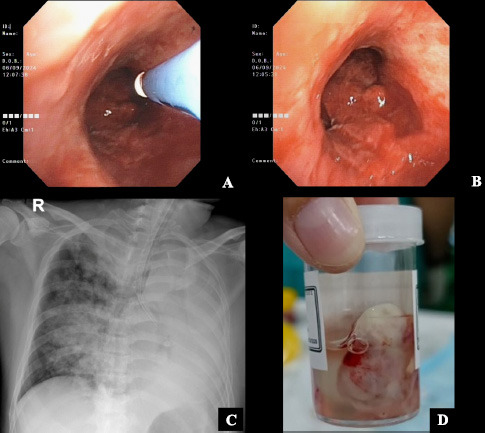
Intraoperative and postoperative findings following bronchoscopic intervention: (A) Argon plasma coagulation (APC) applied to control tumor bleeding; (B) Intentional endobronchial blood clot for tamponade; (C) Post-procedure chest X-ray showing complete left lung atelectasis, right lower lobe opacities, and double-lumen endotracheal tube placement; (D) Gross appearance of the tumor tissue obtained using a cryoprobe biopsy.
